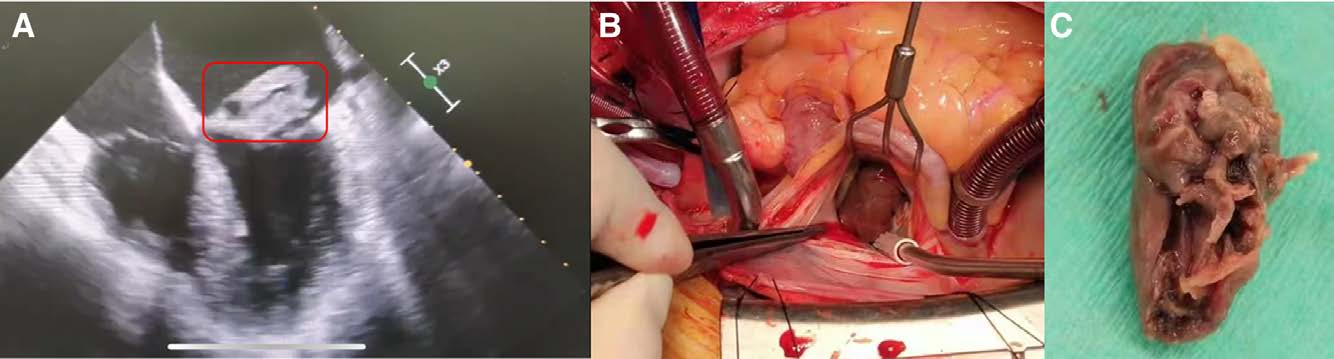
Clinical information of infective endocarditis. (A) Transesophageal echocardiography showing a 22 × 15 mm ectogenic and hypermotile mass on the left atrial mitral valve leaflets. (B) An intraoperative picture of mitral biological valve replacement under general anesthesia. (C) Vegetation in mitral valve.
